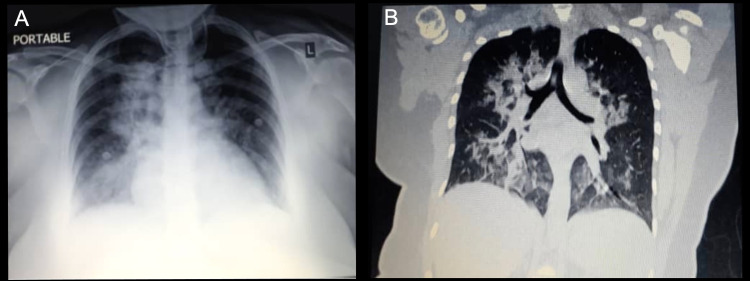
Imaging studies of the 39-year-old mother performed five hours after delivery when the mother developed acutely worsening dyspnea. (A) Chest radiograph and (B) computerized tomography scan revealed bilateral patchy airspace opacities.
